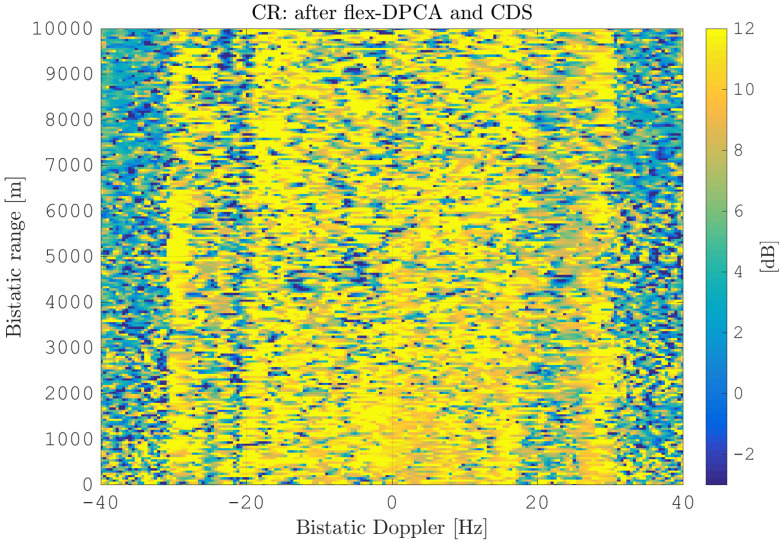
Cancellation ratio between range-Doppler maps after DPCA application: after flex-DPCA (Figure 12) and CDS (Figure 14).
